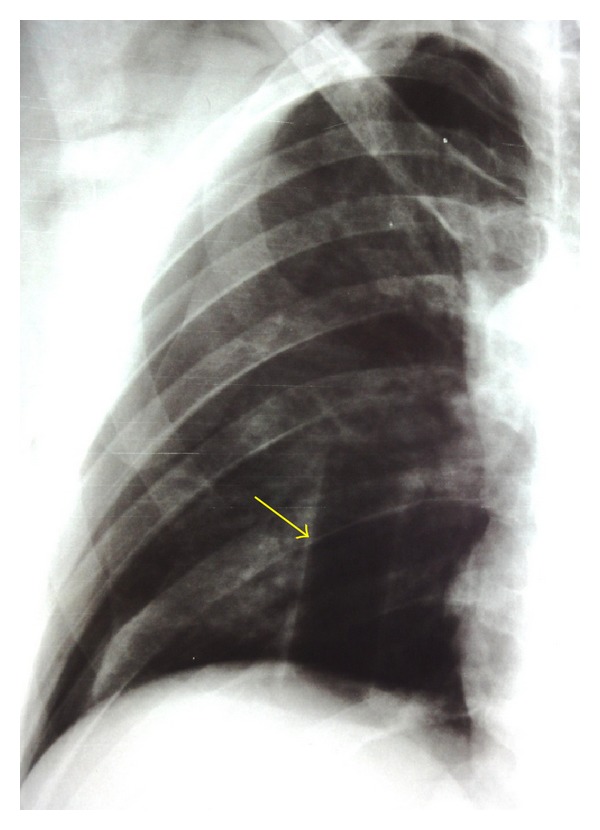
Chest X-ray showing a foreign body into the right thoracic cavity (yellow arrow).
